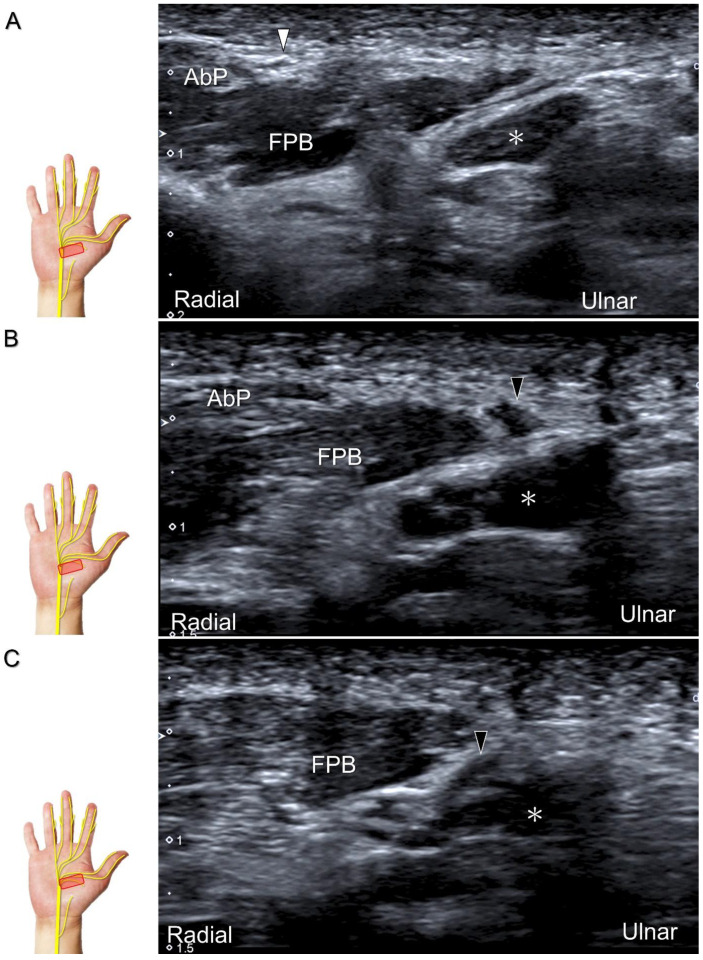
Sonographic imaging (short-axis view) shows the segment (A) distal to the neuroma of the recurrent motor branch of the median nerve (B) and the proximal segment (C). Asterisk: median nerve; white arrowhead: recurrent motor branch of the median nerve; black arrowheads: neuroma. FPB: flexor pollicis brevis muscle; AbP: abductor pollicis brevis muscle.
